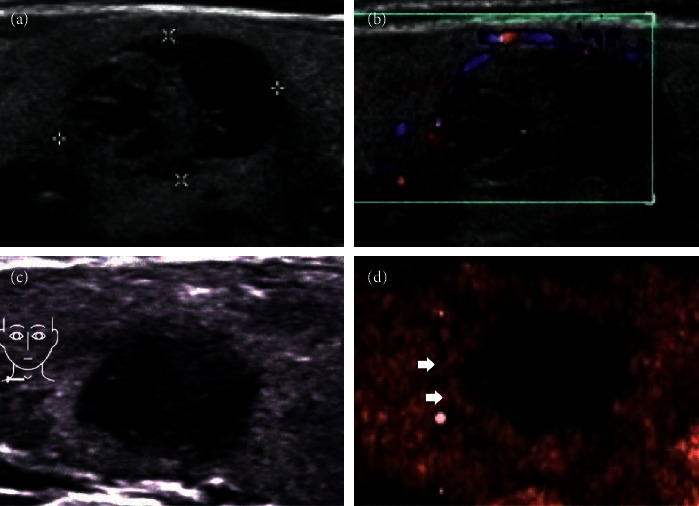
(a, b) A 52-year-old female patient with well-defined cystic and solid nodules in the thyroid gland, and the maximum diameter of the nodules was 17 mm. Color Doppler flow imaging showed blood flow signals in the peripheral and internal solid parts. (c) Three years later, the nodule became smaller, the maximum diameter of the nodule was 9 mm and the margin of the nodule was irregular. (d) CEUS showed no enhancement in the nodules. Short arrows showed annular enhancement at the edges of the nodules. A clear boundary of the nodules could be seen by careful observation.
